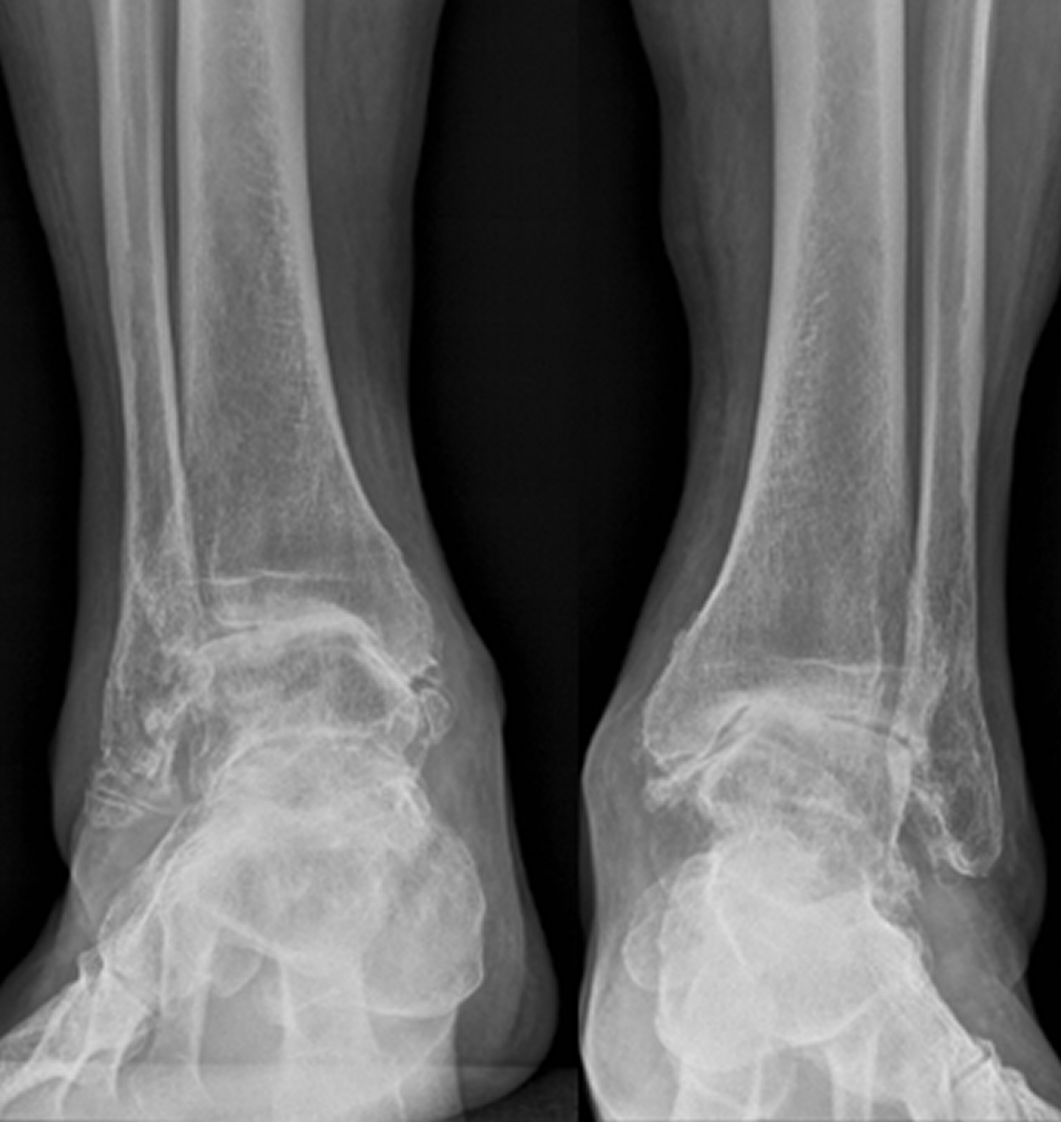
Preoperative anterior radiographs of the ankles. Advanced ankle osteoarthritis with severe varus malalignment on both sides
